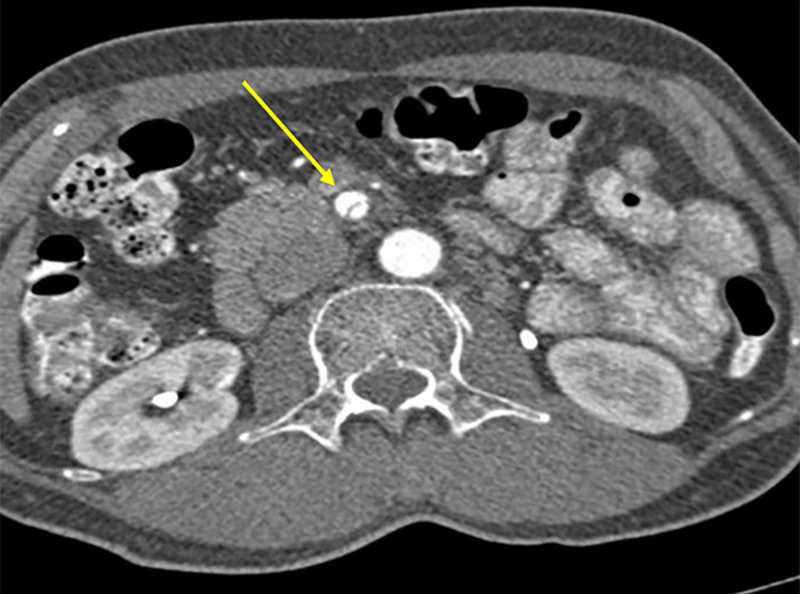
CT aortogram (axial image) demonstrating dissection of the SMA. SMA, superior mesenteric artery
